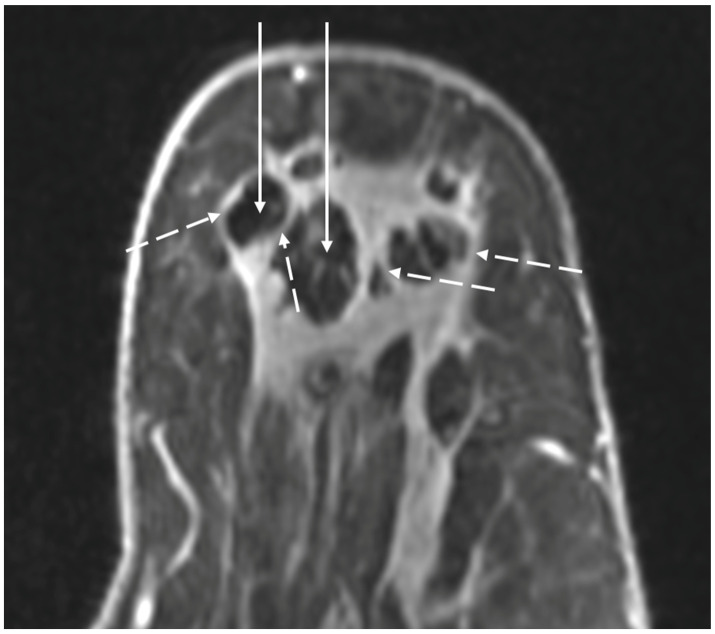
MRI images showing spherical rim enhancement (broken arrows) surrounding relatively avascular cryoablation zones of necrosis (solid arrows).
